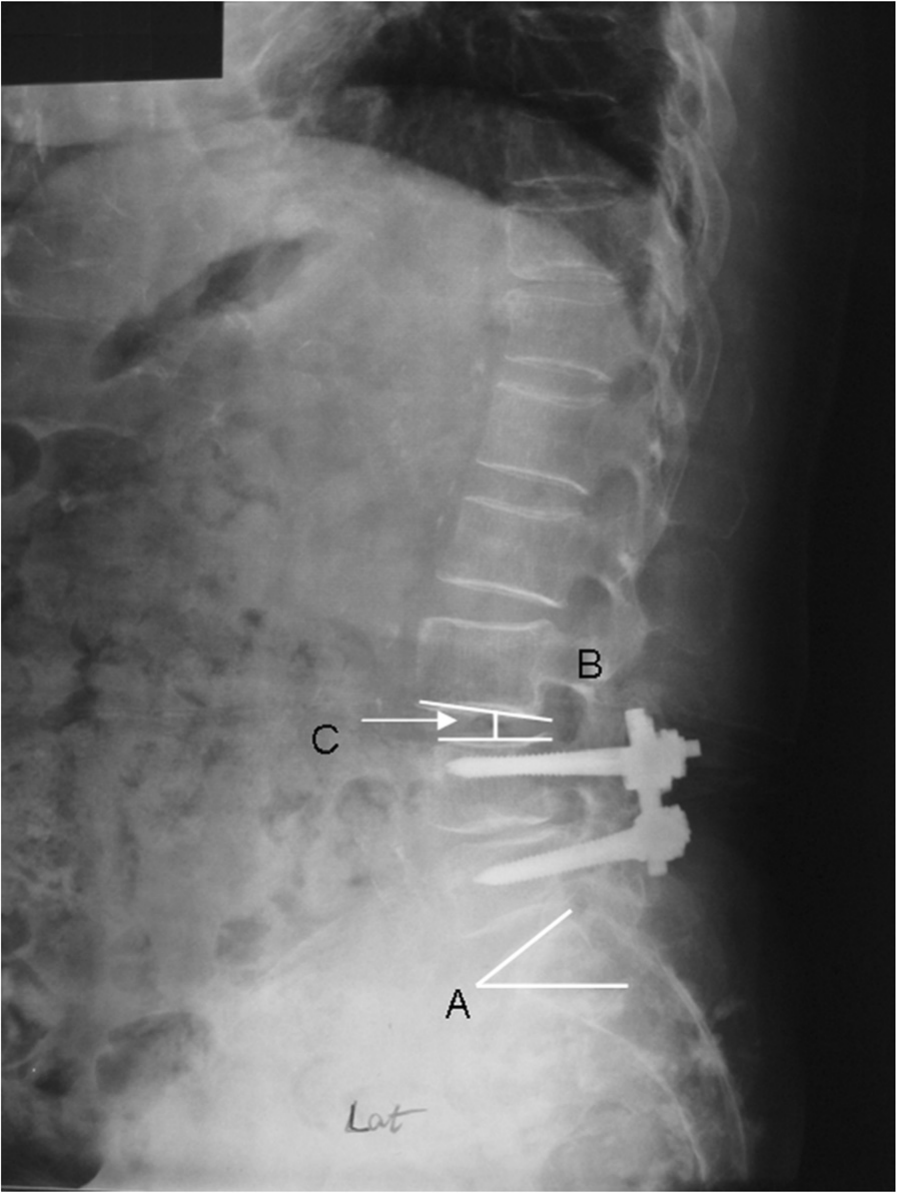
Radiographs assessed for slope angle, disc angle, and disc height. Radiographs assessed for S1 sagittal slope angle (A), adjacent disc angle (B), and adjacent disc height (C).
